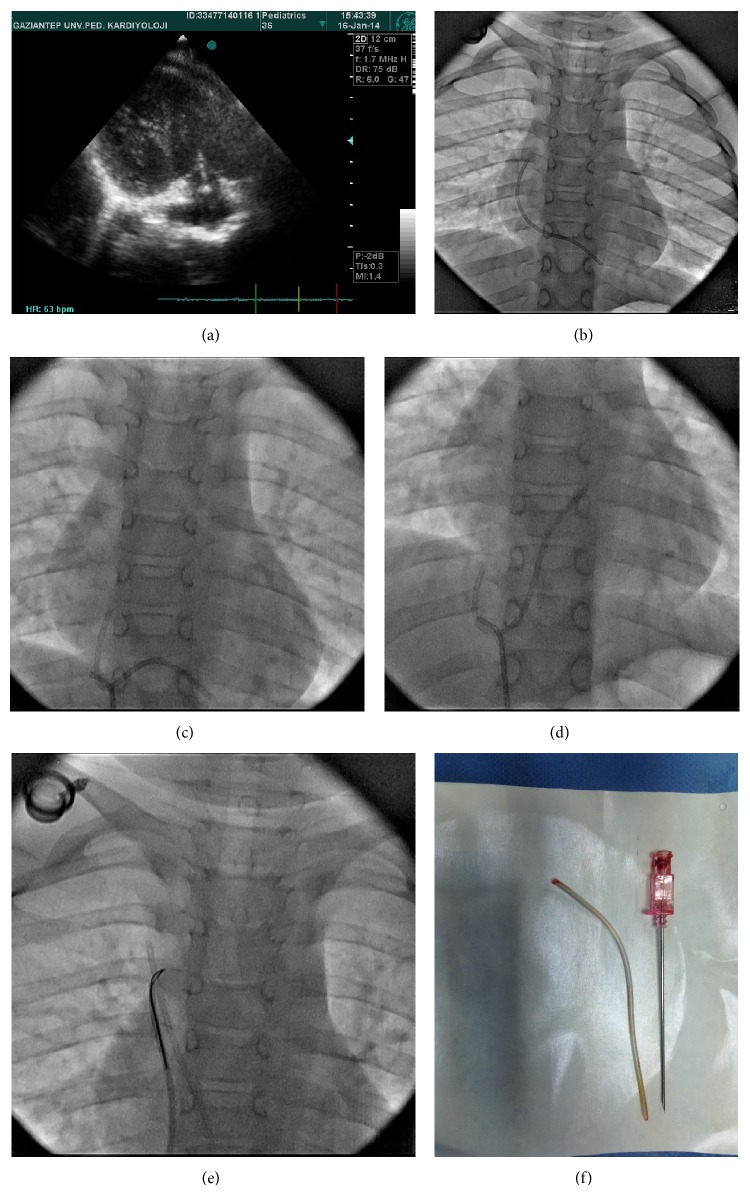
(a) Echocardiographic and (b) angiographic images of a catheter extending to the right ventricle apex from the right atrium roof in a 6-year-old child. ((c), (d), and (e)) Images of its retrieval using a Judkins right catheter as a hook to snare the catheter are shown. (f) The embolized port catheters.
